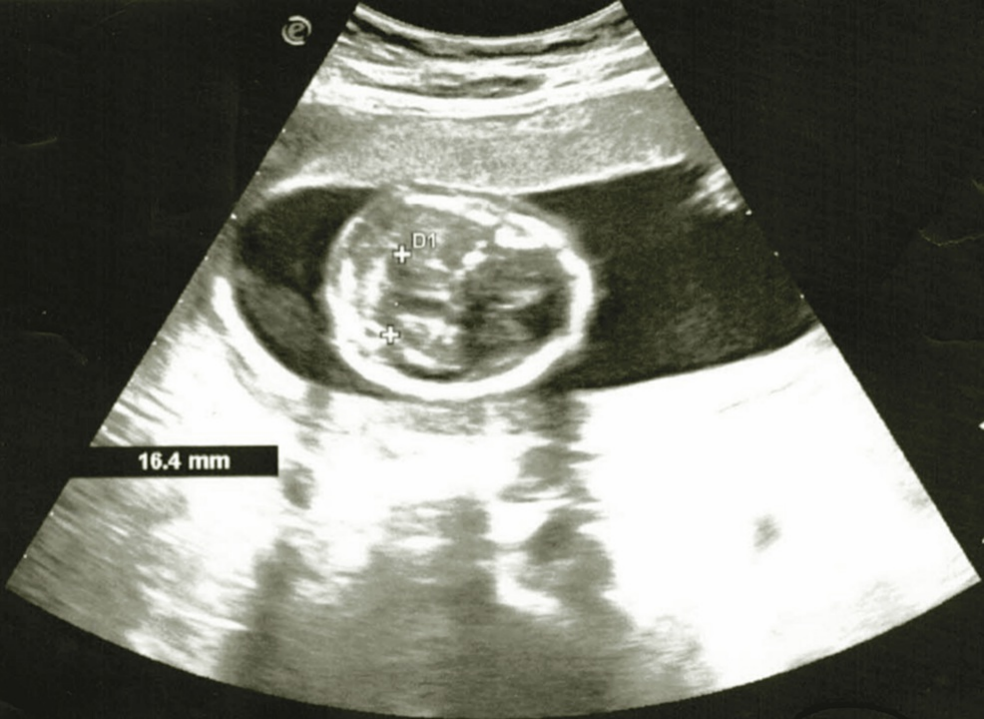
Ultrasound picture of the VPD: 40.0 mm (reference limits: 32-47 mm) VPD, verified parietal diameter
